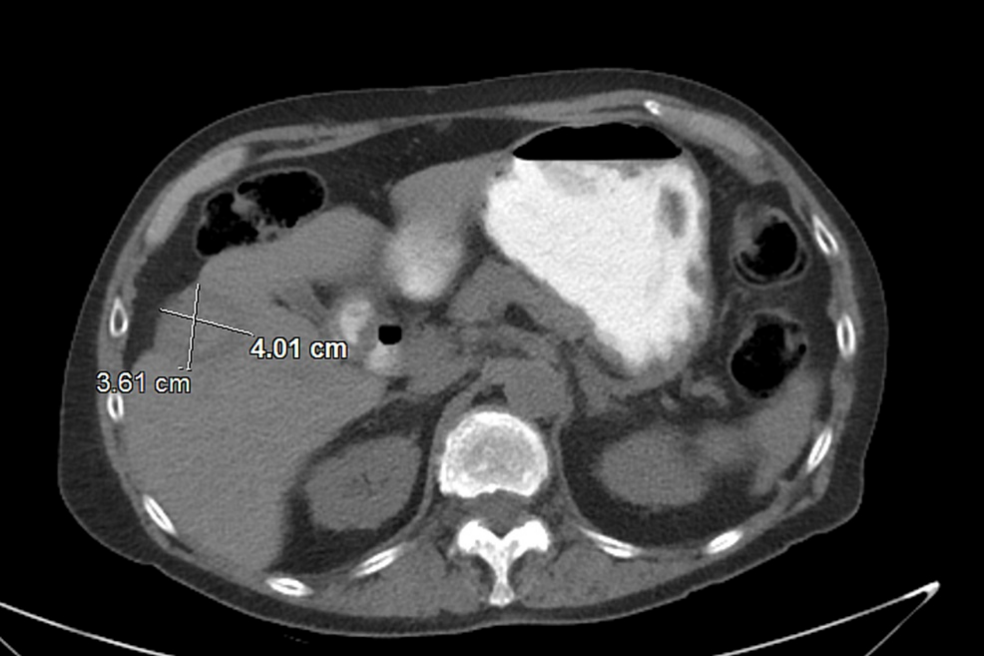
CT scan 48 months after liver metastasis and 18 months after MWA. CT scan showed treatment effects of an ill-defined hepatic mass measuring 4.0 cm without new liver lesions. MWA: microwave ablation, CT: computed tomography
